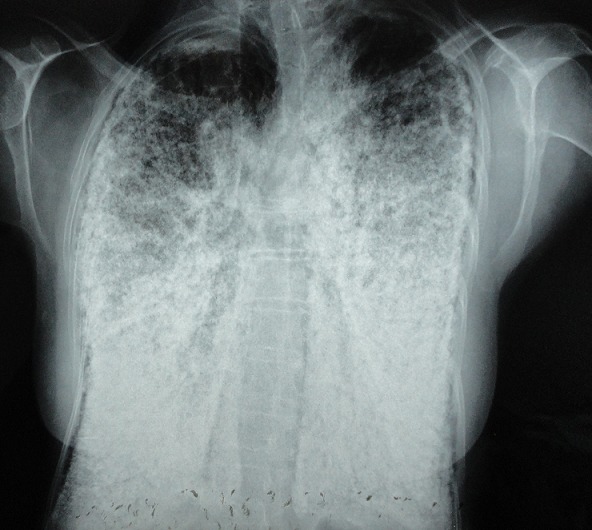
Radiographie thoracique de face actuelle montrant le même aspect plusieurs années après
